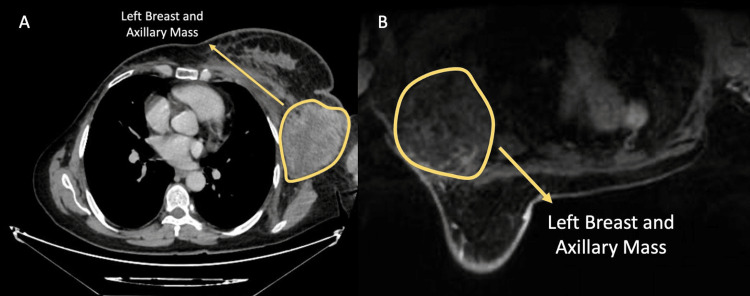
Post-contrast CT and MRI images demonstrating a left axillary mass A) Post-contrast axial CT image demonstrating a left axillary mass B) Post-contrast axial T1-weighted breast MRI image demonstrating a left axillary mass
